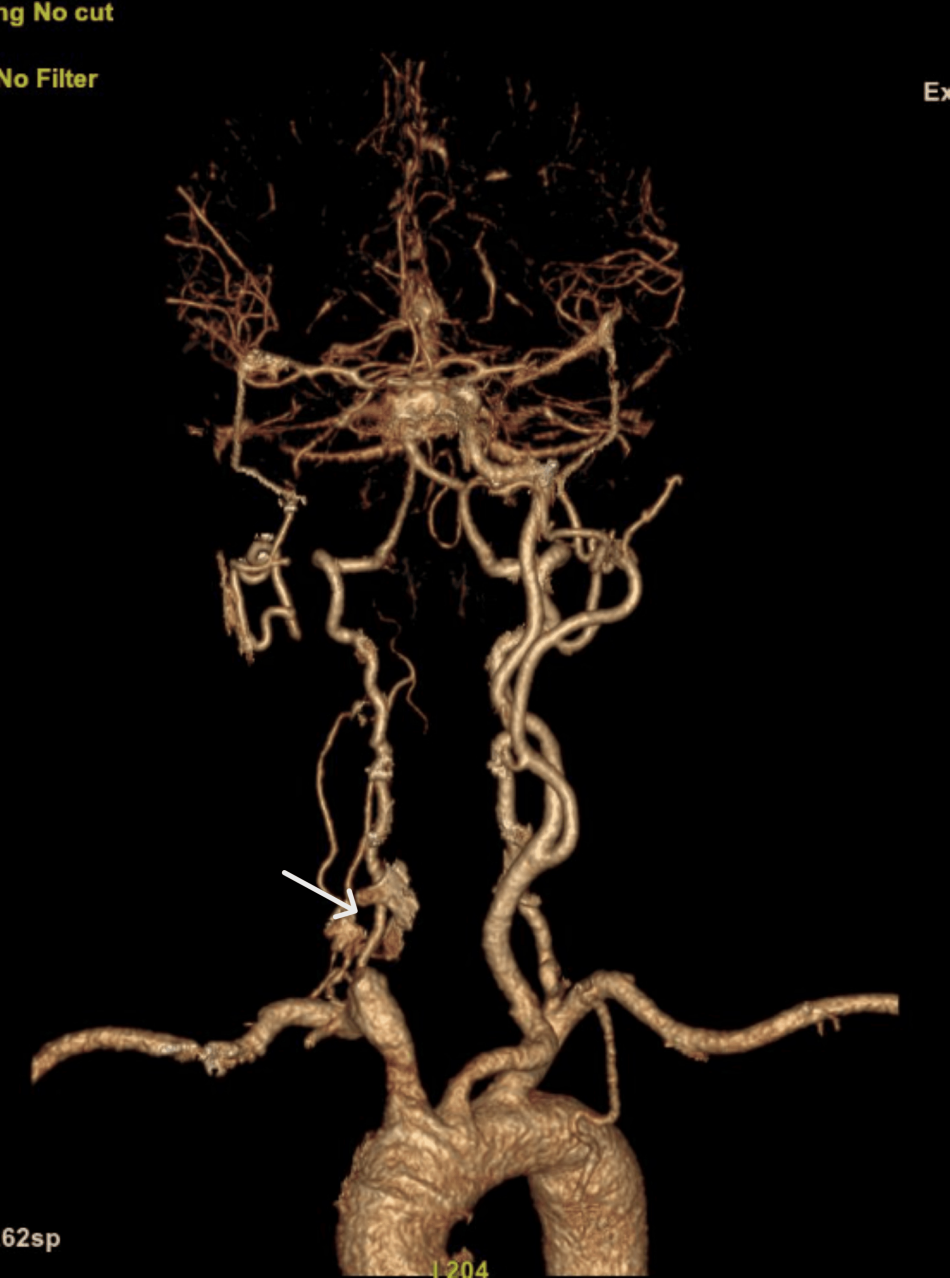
CT brain with angiogram showed complete occlusion of most of the right CCA, ICA (C1 to C6 segments), and ECA. Bilateral ACA and MCA are patent and show normal contrast flow. Posterior circulation, including bilateral vertebral arteries, is patent. The white arrow shows complete occlusion of the right CCA. CCA: common carotid artery; ICA: internal carotid artery; ECA: external carotid artery; ACA: anterior cerebral artery; MCA: middle cerebral artery
